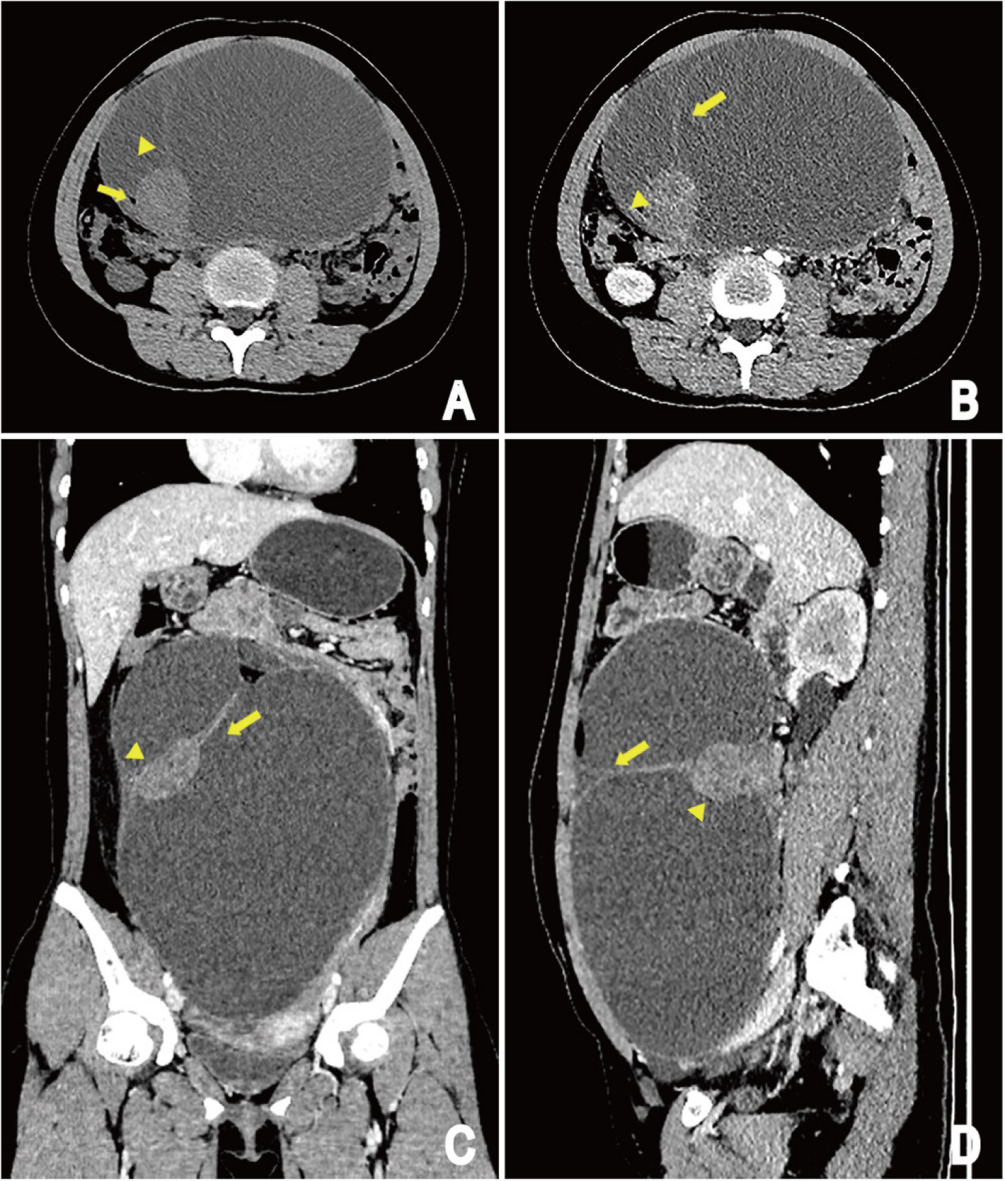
CT images of patient: Unenhanced CT image shows a large oval cystic-solid mass in abdomen pelvic cavity, originating from the left ovary, the margin of the mass is clear and the cystic part is the main component in the mass; The round-like solid component shows inhomogeneous density shadow, CT value is about 39 HU, with smooth edge yet. The cystic part has internal division change (arrowhead) and fatty lesions (arrow) (a). The tumoral solid components (arrowhead) and its internal division (arrow) could be seen intensified from slight to moderate on contrast-enhanced CT images compared with those on precontrast images, and the solid component shows heterogeneous enhancement. Adjacent organs such as the intestine, the uterus displaced by compression (b, c and d). (b) Arterial phase of contrast enhancement image; (c and d) portal phase of contrast enhancement image
